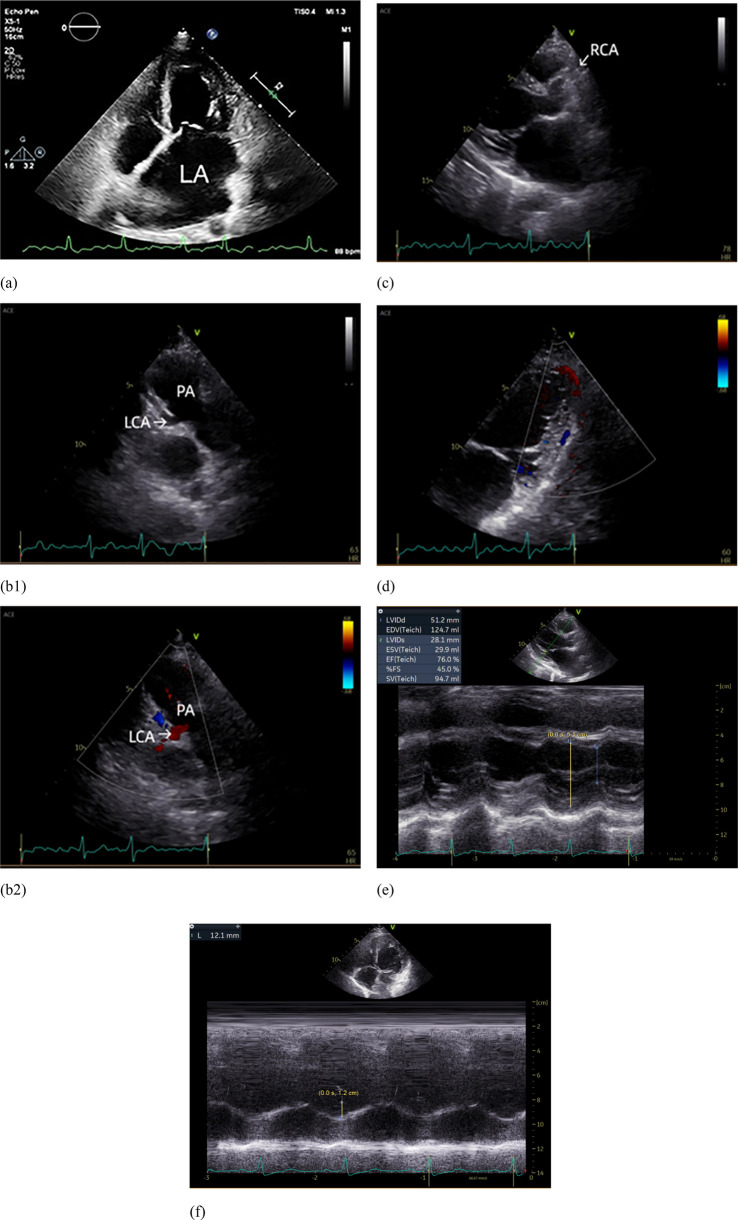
Echocardiograph. (a) The left atrium was markedly enlarged; (b1) The left coronary artery (LCA) originates from the pulmonary artery (PA); (b2) Blood flow from LCA to PA; (c) The right coronary artery (RCA) originates from the right coronary sinus and is dilated. (d) Intramyocardial visualization of blood flow signals between the LCA and RCA. (e) Dilatation of the left ventricle. (f) Reduced tricuspid annular plane systolic excursion (TAPSE).
